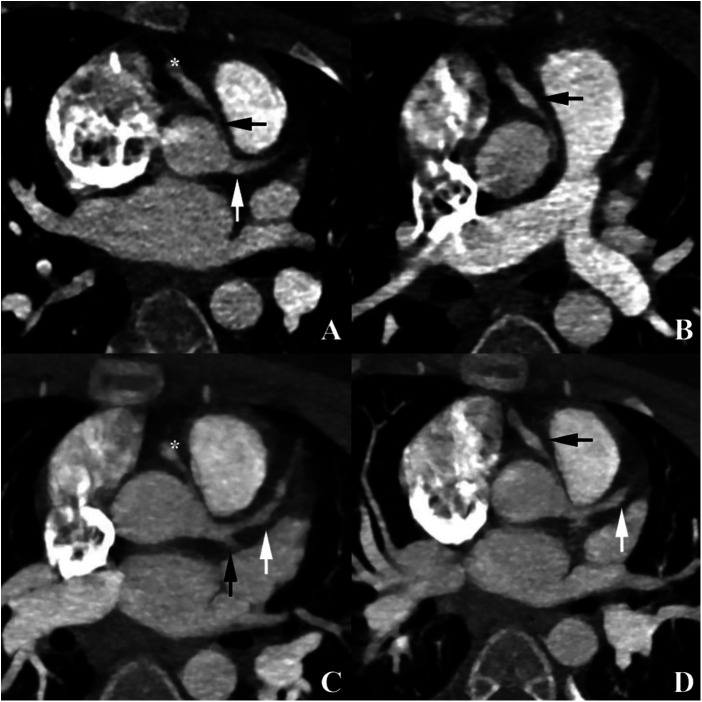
Cardiac computed tomography. Anomalous origin and course of the right coronary artery (RCA) (A) Axial image demonstrates an interarterial course of the RCA (black arrow) with proximal compression and aneurysmal dilatation (asterisk). A single coronary artery (white arrow) originates from the left sinus of Valsalva and divides into the left coronary artery and RCA. (B) Visualization of aneurysmal dilatation of the RCA (black arrow). (C) MIP reconstruction shows pronounced aneurysmal dilatation of the RCA (asterisk) and left anterior descending artery (LAD) (white arrow). The origin of the circumflex artery (Cx) is indicated by a black arrow. (D) MIP reconstruction demonstrates aneurysmal dilatation of the RCA (black arrow) and LAD (white arrow).
